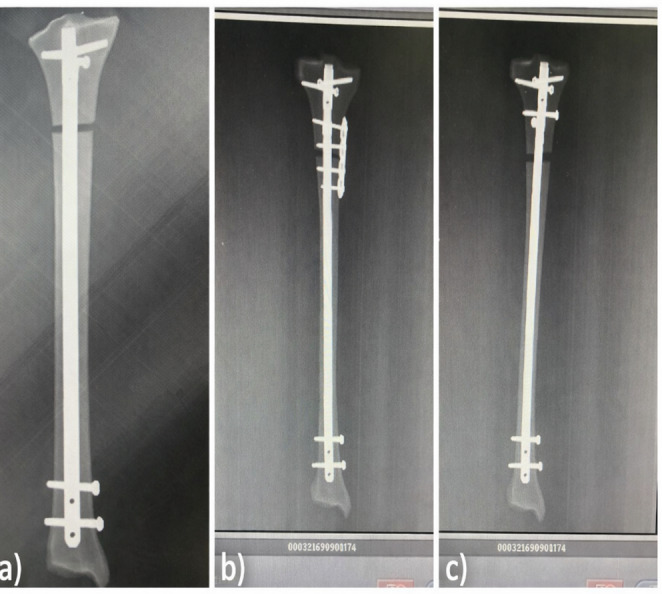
X-ray view (anterior-posterior radiography) of synthetic tibial bone models. a Intramedullary nail (IN). b Intramedullary nail (IN) + plate. c Intramedullary nail (IN) + Poller screws
